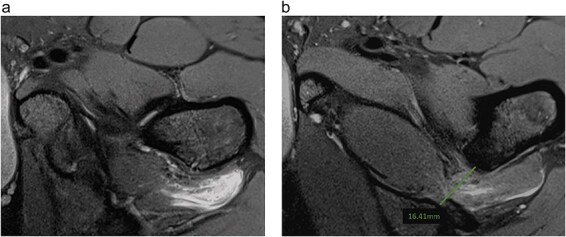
Axial T1-weighted fat-suppressed MRI images after intravenous injection of gadolinium demonstrate (a) enhancement surrounding quadratus femoris muscle (*) (b) EIF left hip measuring 16 mm.
